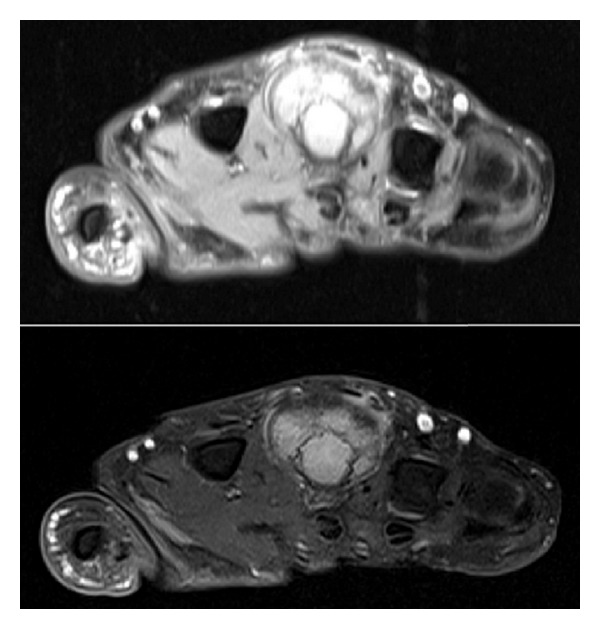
Axial T1/T2-weighted postoperative MR images of the third metacarpal demonstrate a somewhat mushroom-shaped, pedunculated lesion arising from the dorsal aspect of the third metacarpal with an internal signal similar to that of the underlying marrow.
